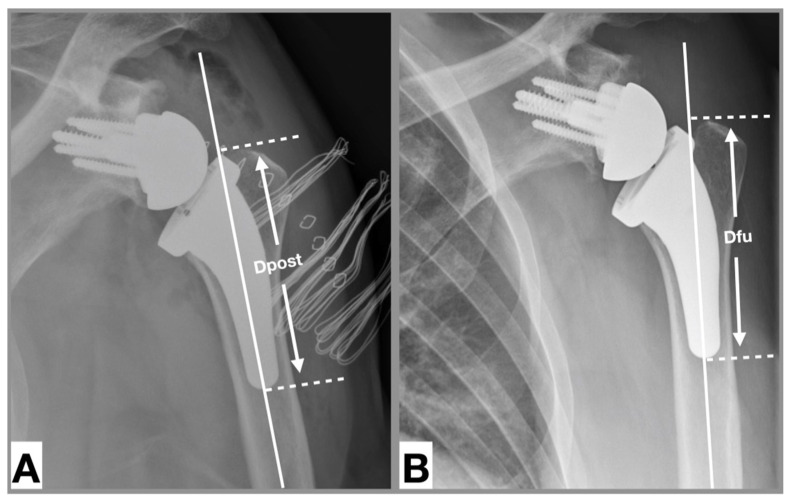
Measurement of subsidence [33]. Anteroposterior radiograph of a left shoulder. (A). Postoperative, (B). At 12 months follow-up. Shaft axis (solid white line), tip of tuberosity (white dotted line perpendicular to shaft axis), distal end of trabecular metal (white dotted line perpendicular to shaft axis). Subsidence: Distance between white dotted line at follow-up minus distance between white dotted line postoperative. Distances were calibrated based on the size of the glenosphere.
